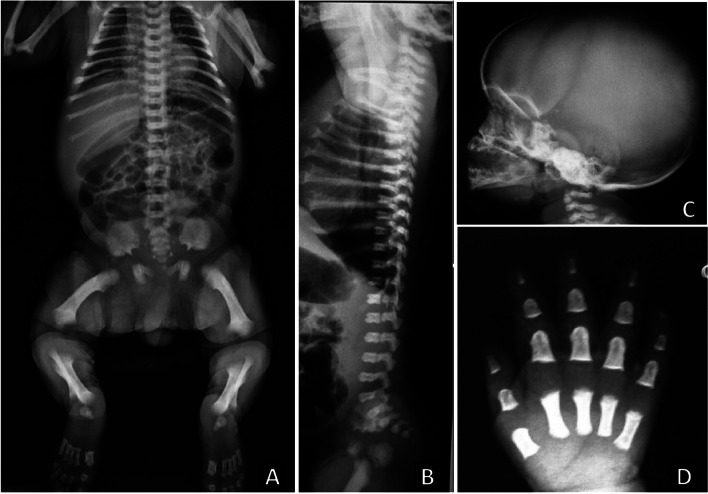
Radiographic images of achondroplasia in the newborn period. A Generalized platyspondyly leading to an increased height of the intervertebral spaces. The pelvis shows a squared configuration with a horizontalized acetabular roof and narrowness of the sciatic notch. Pubis and mainly ischia are broad. The long tubular bones are shorter with metaphyseal irregularity and the fibulae are greater than tibia. Note the oval-shaped lucent appearance of the proximal femora. B In the lateral view, it is also evident an increased lumbar lordosis. C The cranium is large and both frontal and occipital regions are prominent. D The hand show shortening of all tubular bones – metacarpal and phalanges
